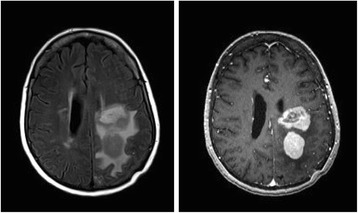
Brain MRI (April 2010): axial FLAIR (left), axial T1 post contrast (right) reveal progression of nodular enhancement of the persisting left-sided lesions
